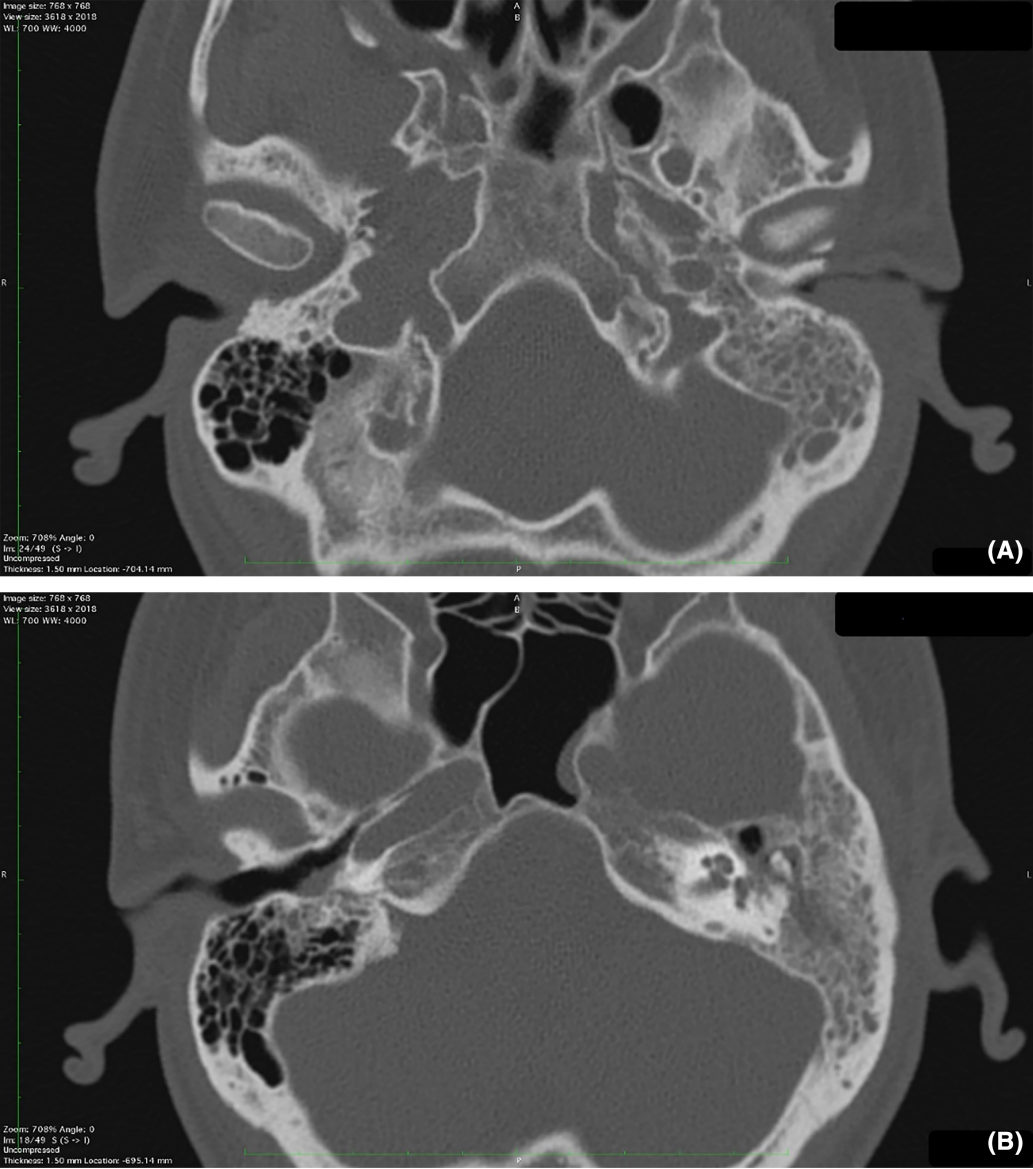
Head computed tomography. Computed tomography reveals a complete homogenous left‐sided and partial right‐sided opacification of the mastoid cells and the middle ear with a relatively intact bony structure. A significant narrowing of the left external auditory canal (A) is shown, compared to the right external auditory canal (B).
